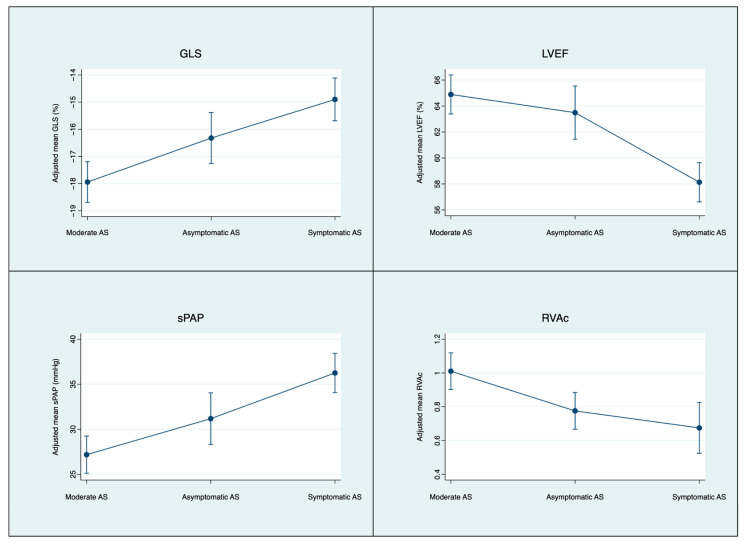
Trajectories of key echocardiographic parameters (LV GLS, LVEF, sPAP and RVAc) across the clinical course of AS. Time points correspond to the predefined echocardiographic assessment. Solid lines represent model-based estimated means derived from mixed-effects models with a patient-level random intercept to account for repeated measures; vertical bars indicate 95% confidence intervals. LV GLS is reported as signed values (more negative indicates better systolic function). RVAc was defined as TAPSE/sPAP and was treated as missing when sPAP could not be estimated. Abbreviations: AS, aortic stenosis; GLS, global longitudinal strain; LVEF, left ventricular ejection fraction; sPAP, systolic pulmonary artery pressure; RVAc, right ventricular–arterial coupling.
